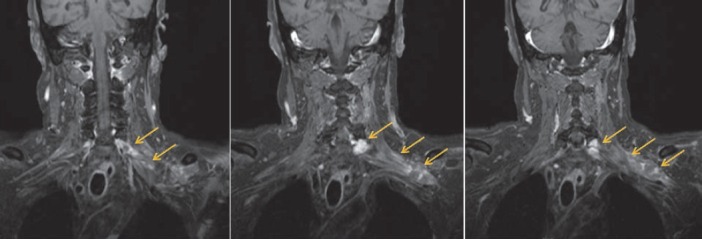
Coronal fat-suppressed, contrast-enhanced T1W MRI showed enlargement and enhancement of the left C7, C8 nerve roots and brachial plexus
